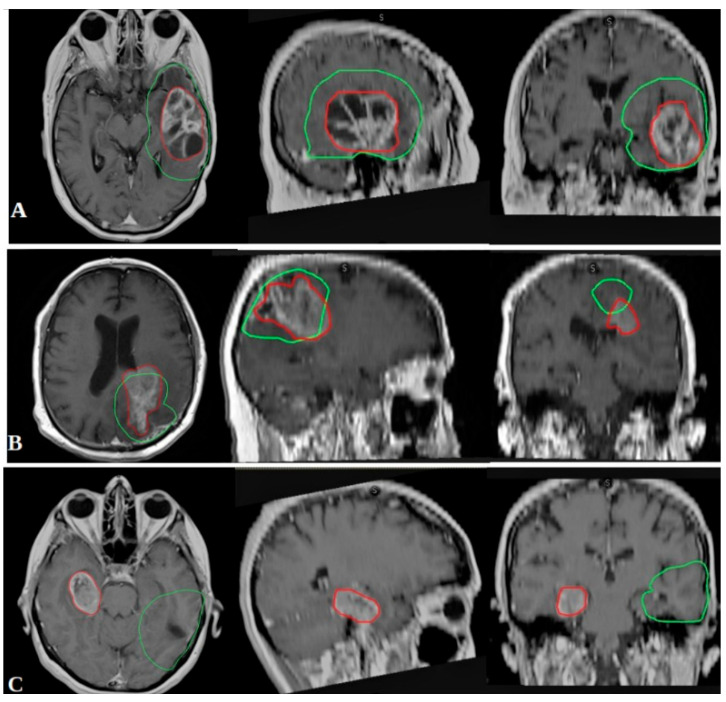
Pattern of relapse classification. Recurrence patterns were evaluated by co-registering follow-up MRI scans with RT treatment plans using RayStation^®^ software (RaySearch Laboratories AB, Stockholm, Sweden) and were categorized based on spatial distribution in relation to the 95% isodose line. Spatial recurrence: (A) in-field; (B) marginal; (C) Out-field. Green: PTV; Red: RELAPSE.
